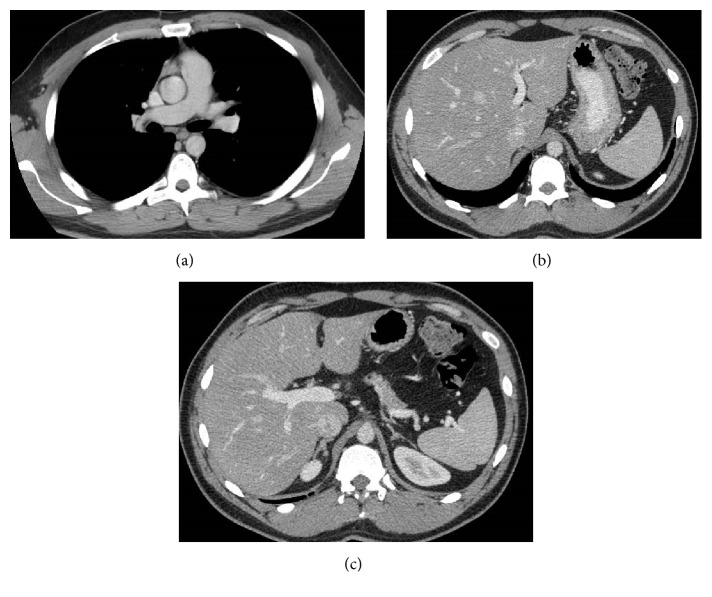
Contrast-enhanced multislice CT of the chest (a) on mediastinal window showed no thoracic lymphadenopathy. Multislice abdominopelvic CT scans (b and c) with intravenous contrast administration showed no significant findings, excluding focal liver or splenic lesions.
